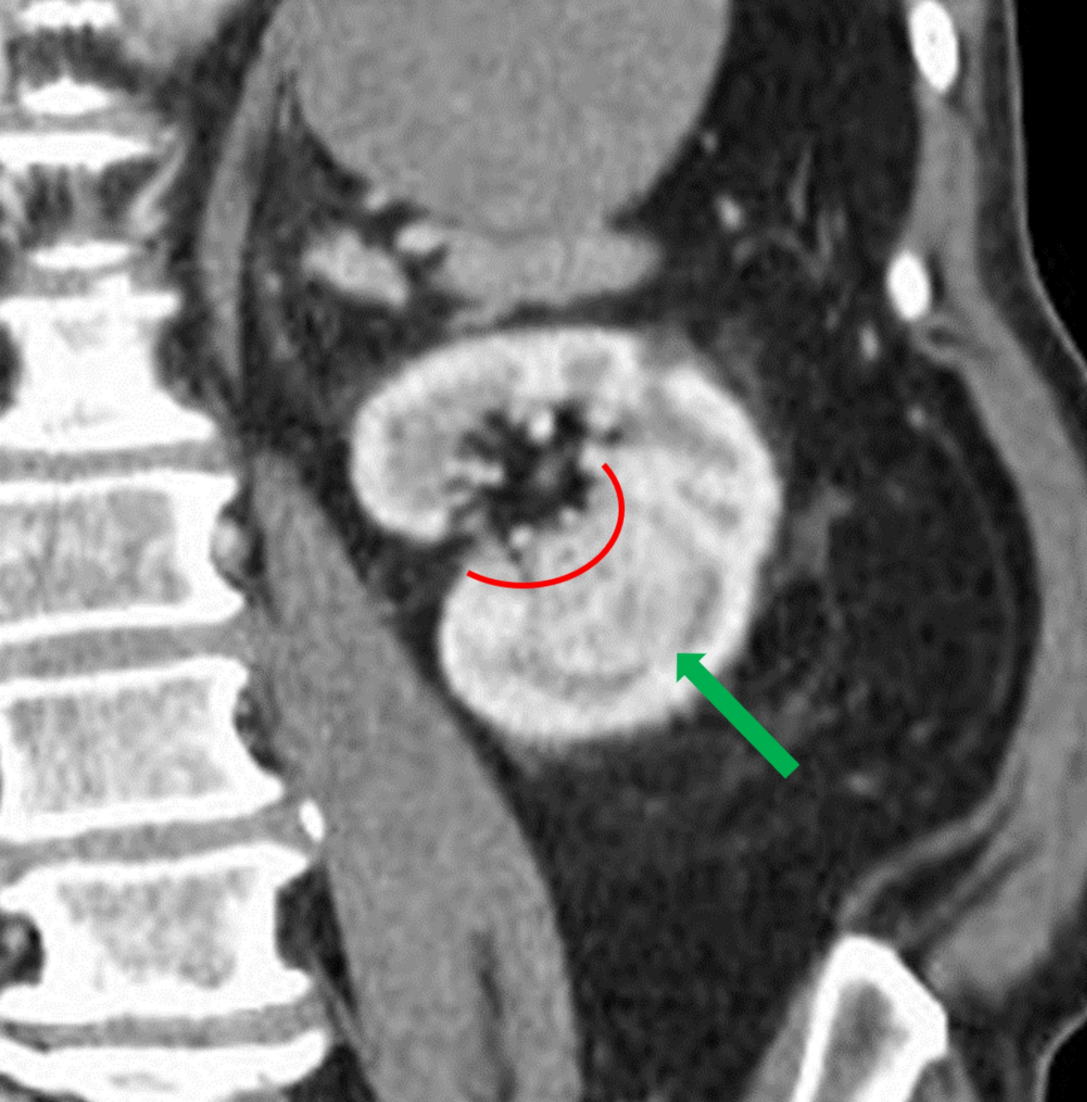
Initial coronal CT showing an incidental renal mass measuring 3.1 cm x 2.6 cm (green arrow) with extension into the renal pelvis (red curved line). Abbreviation: CT, computed tomography.
